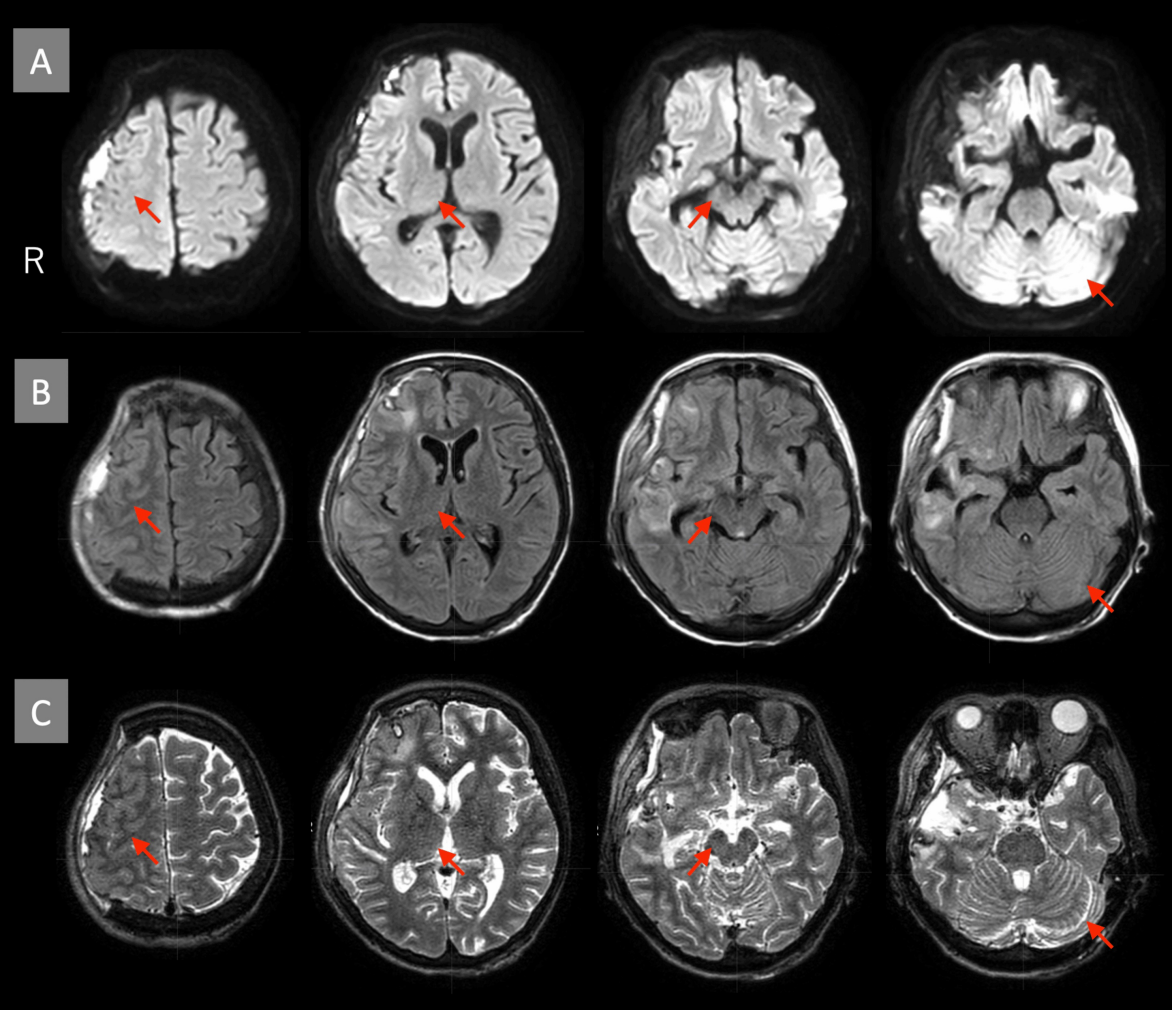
MRI of the head pre-cranioplasty (day 27) (A) Diffusion-weighted image (b=1000 s/mm²). (B) FLAIR image. (C) T2-weighted image. There were no lesions associated with motor, sensory, or coordination functions. The arrows show the right primary motor cortex, thalamus, cerebral peduncle, and left cerebellar hemisphere. MRI: magnetic resonance imaging; FLAIR: fluid-attenuated inversion recovery
